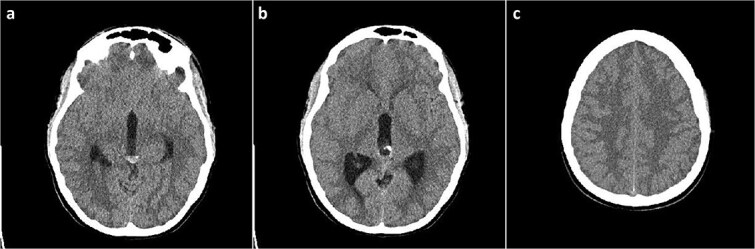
Head CT scan, axial slices. (a) Cystic lesion inside the posterior part of the third ventricle with a hyperdense content in the posterior part of the cyst; (b) probable calcifications in the cyst walls; (c) diffusely obliterated sulci of the convexity as a sign of obstructive hydrocephalus.
